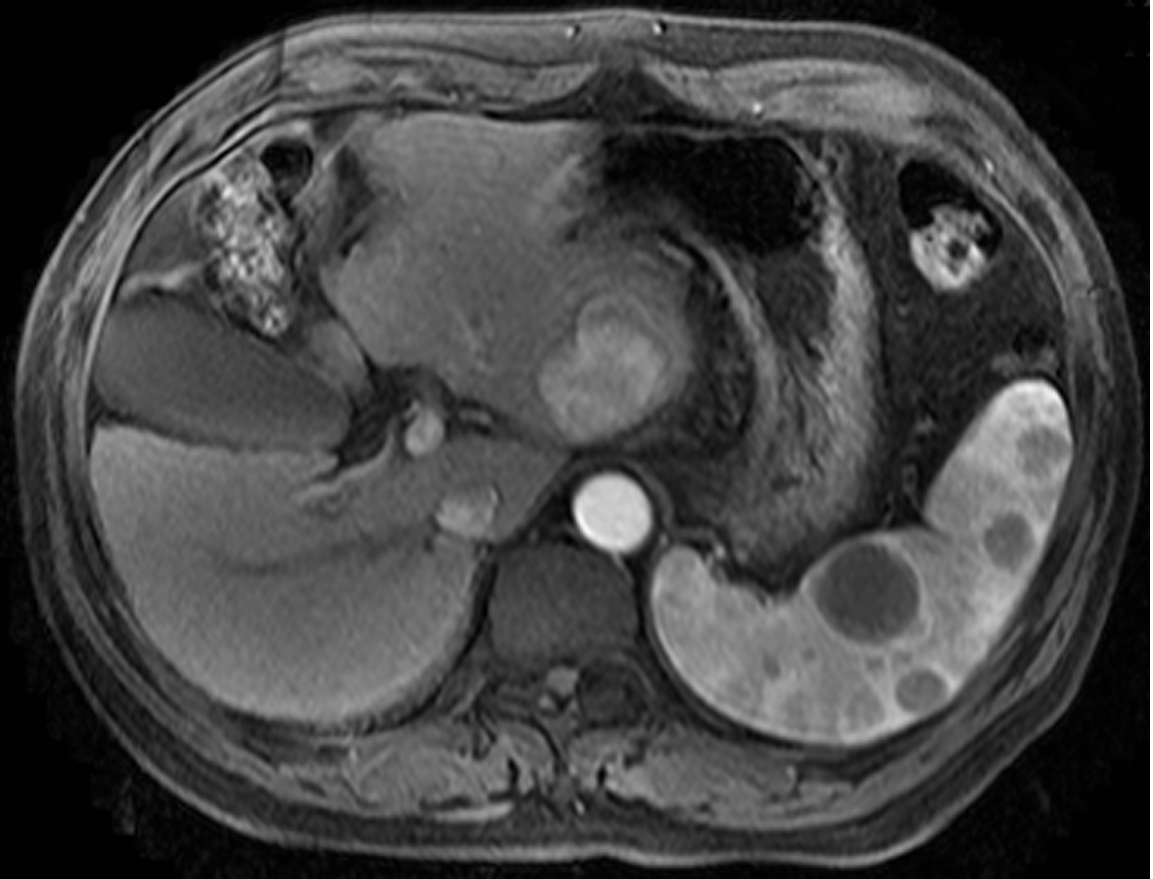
Magnetic resonance imaging showed the tumor in the left lateral segment of the liver, and a multiple, small-to-moderate nodules in the enlarged spleen.
